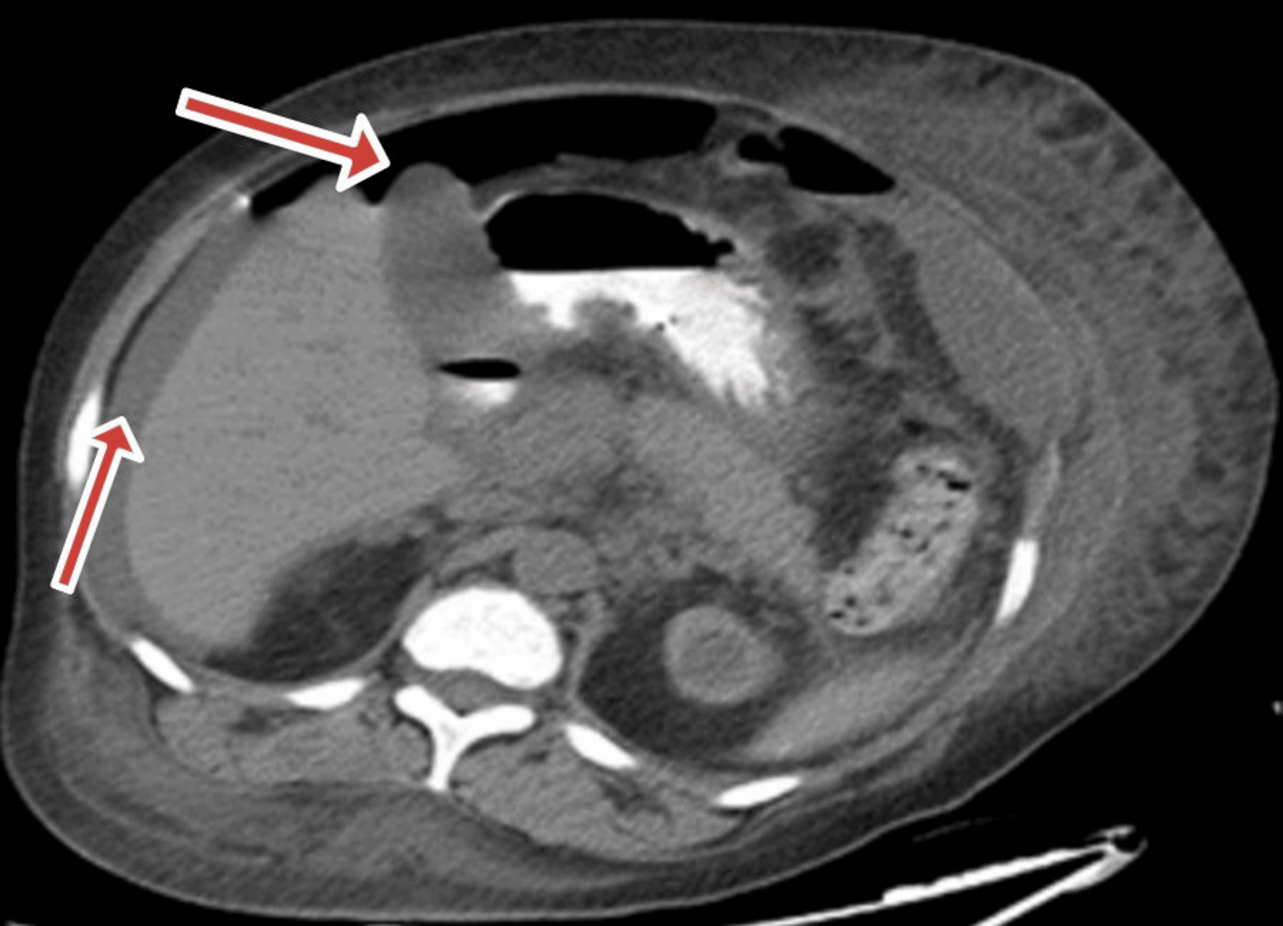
CT abdomen and pelvis showing a small-volume pneumoperitoneum, moderate ascites, mild pleural effusion, and retroperitoneal lymph nodes
